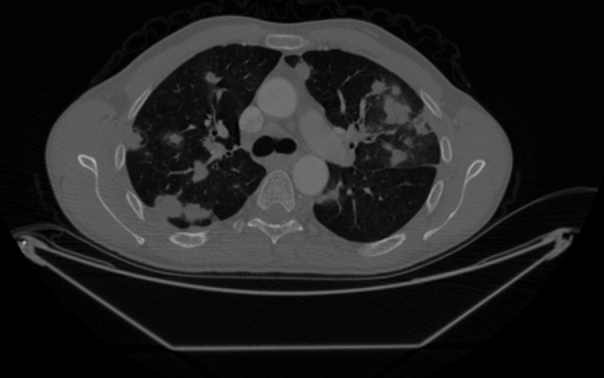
Multiple intraparenchymal and subpleural metastatic nodules in the thorax CT of the first patient.
